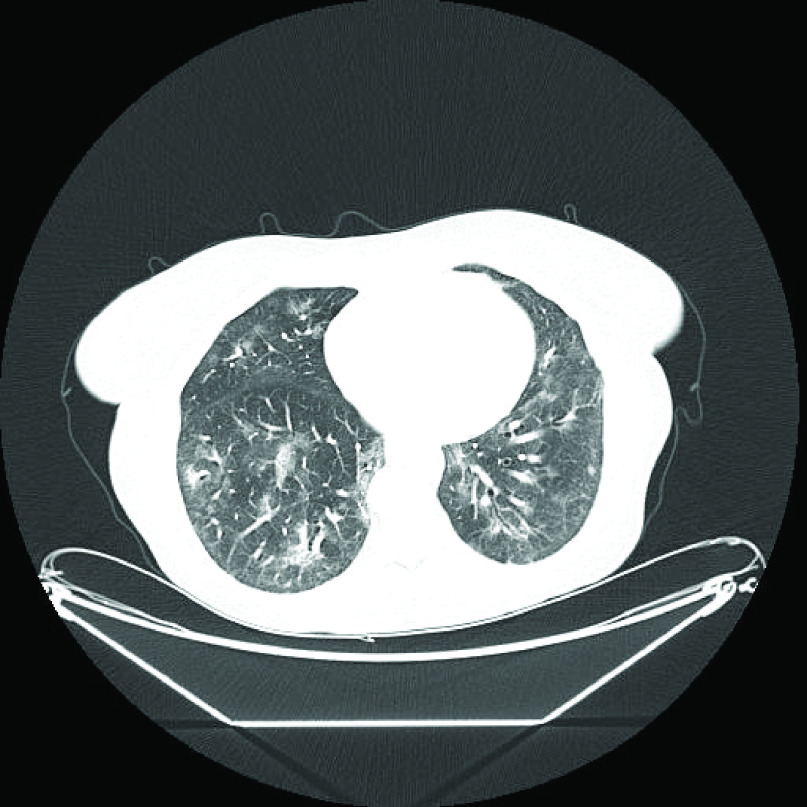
Chest CT performed upon COVID-19 diagnosis showing multiple ground-glass opacities and patchy consolidations in both lungs.
